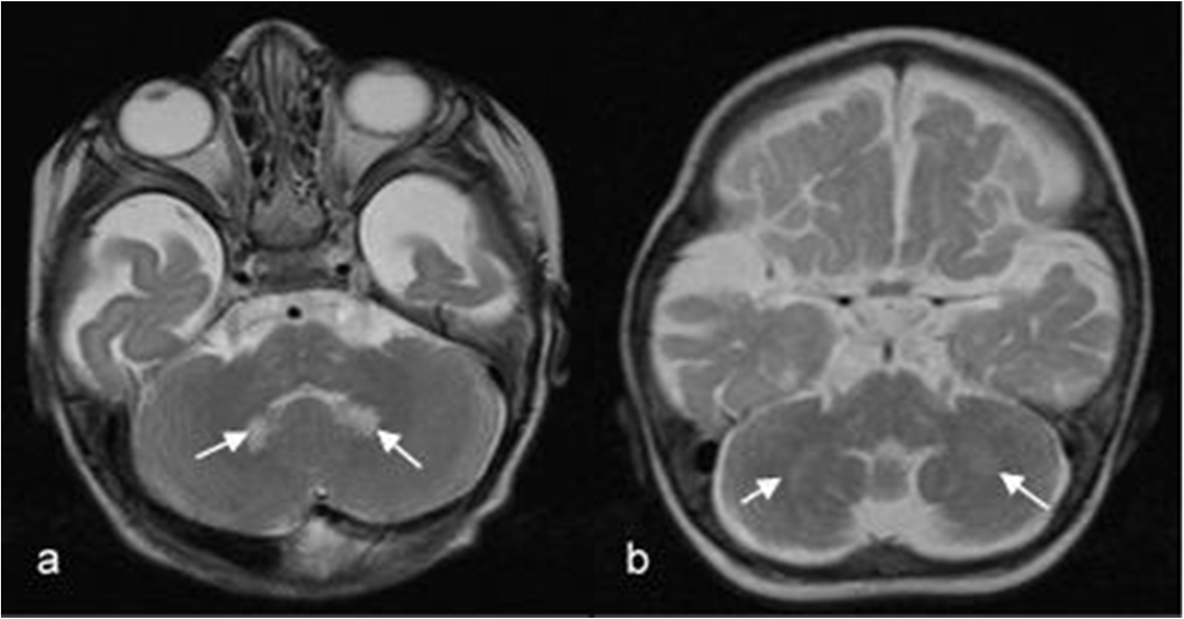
Pattern A1: cerebellar changes in an early-infantile patient during the disease course (onset 4 months). The T2-weighted axial MRI at age 5.5 months shows hyperintensity in the dentate nucleus (a) which has disappeared eight months later (b).
